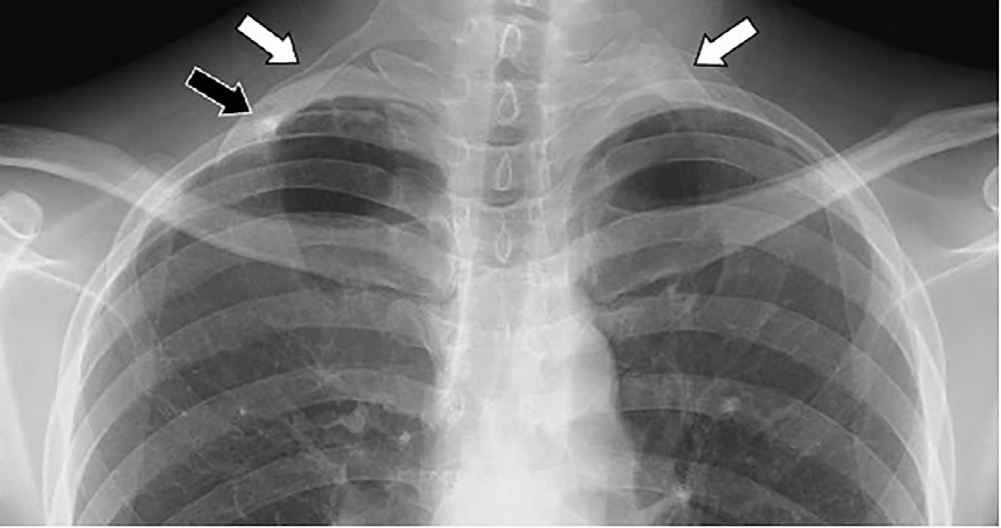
Chest radiography. Bilateral abnormal rib-like bony structures resembling cervical ribs are observed (white arrow). On the right side, focal osteosclerosis is noted at the site of overlap with a caudal bone (black arrow).
